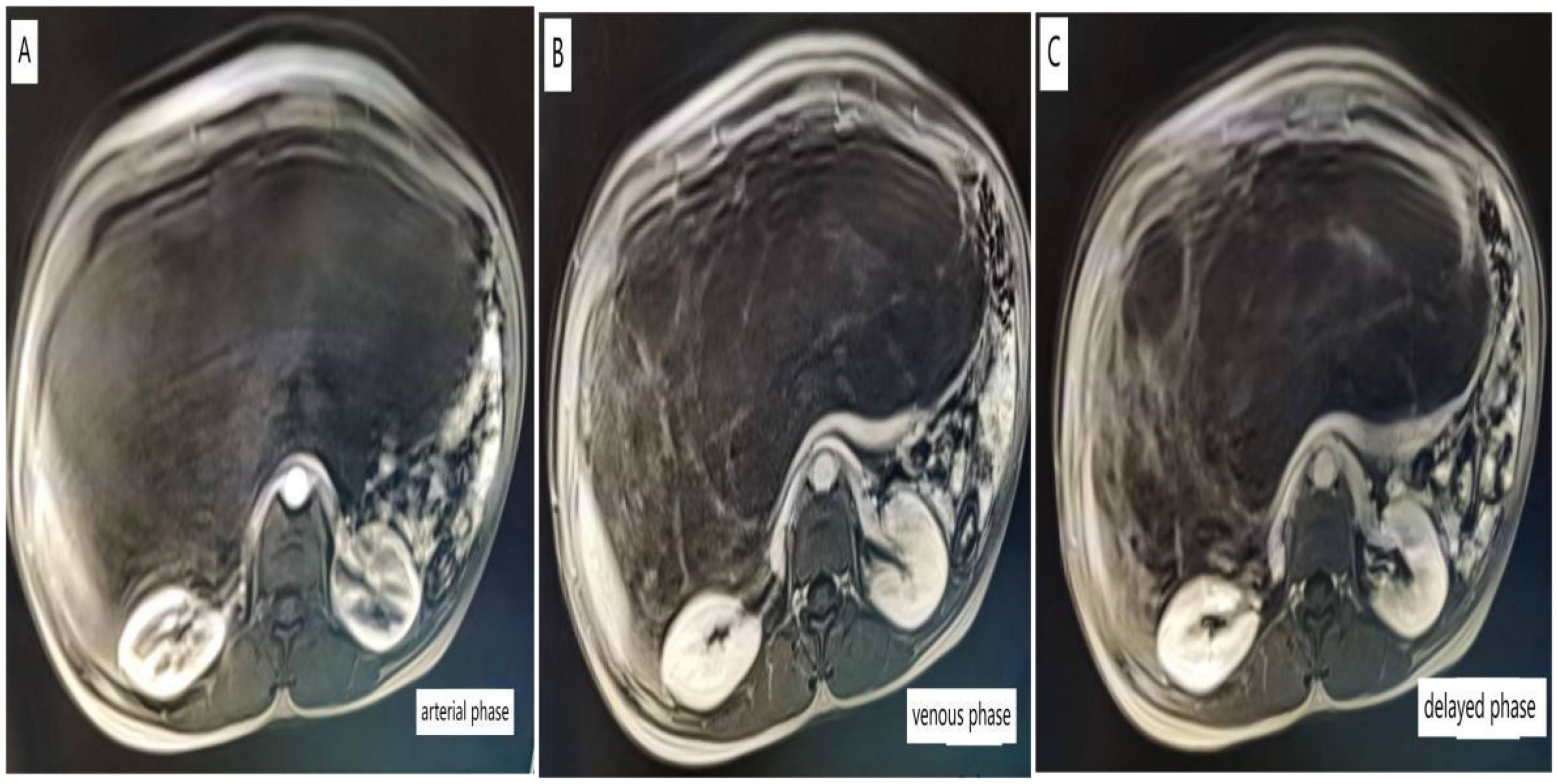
Pelvic-enhanced MRI images of arterial phase (A), venous phase (B), delayed phase (C), and Pelvic MRI revealed huge lesions in the pelvis, which were cystic, uniform, and multiventricular, including multiple compartments.
